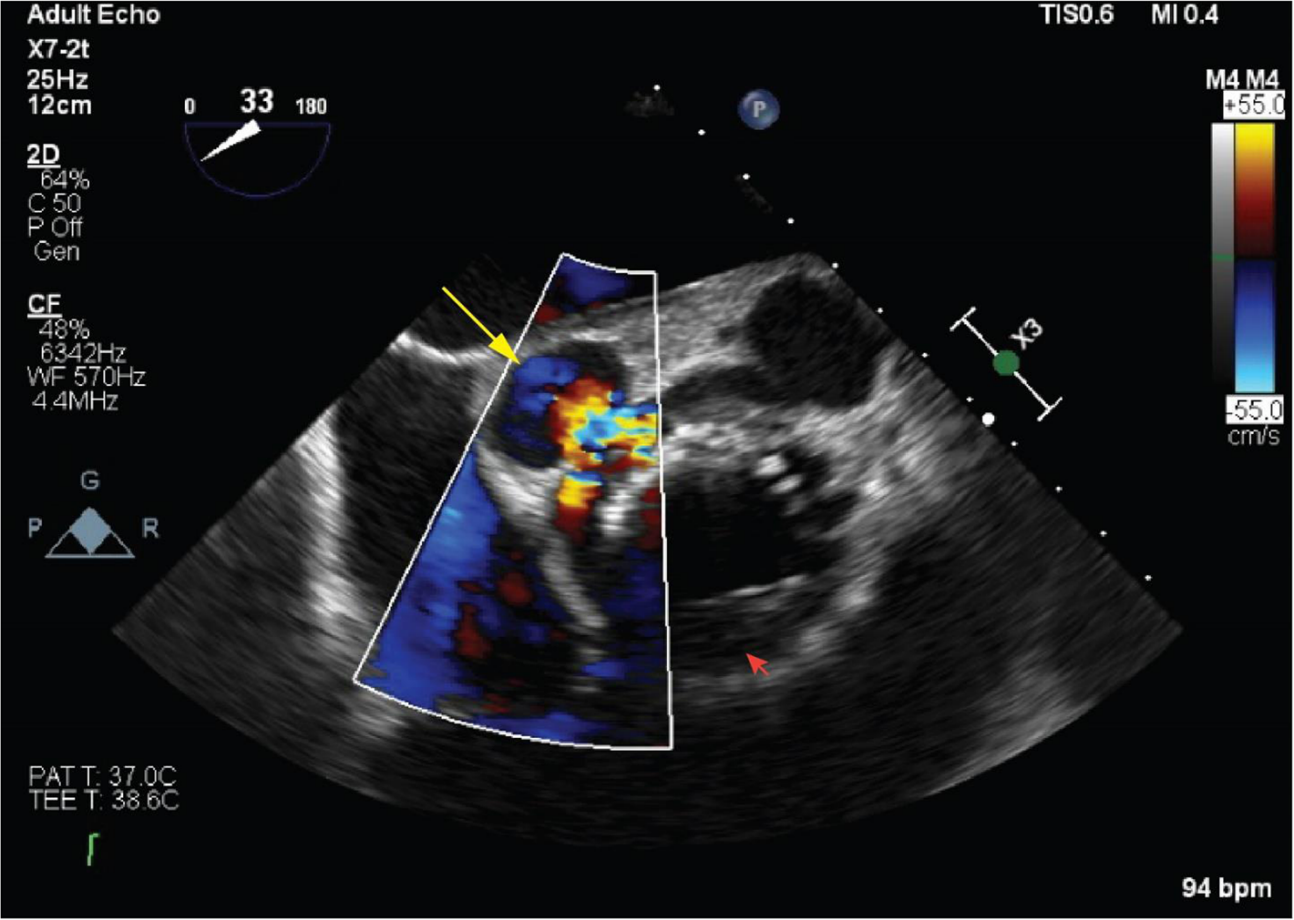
Preoperative echocardiography: Preoperative imaging showing severe aortic regurgitation and pseudo-aneurysms originating from the aortic annulus (yellow arrow). A shallow membrane is protruding from the aortic annulus, fixing the prosthetic aortic valve (red arrow)
